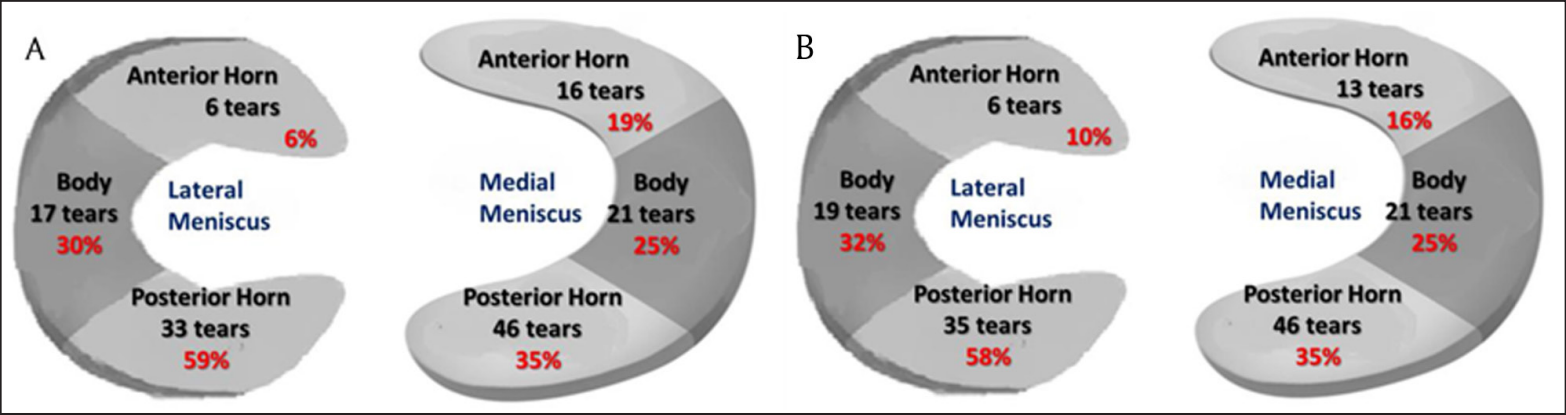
(a) Meniscal tear distribution on MRI (b) Meniscal tear distribution at arthroscopy.
